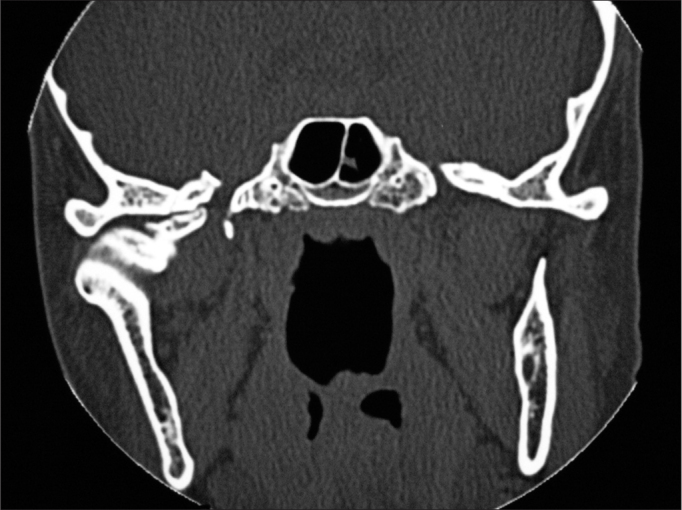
Coronal CT scan: peripheral osteoma in the right-side mandible condyle.
